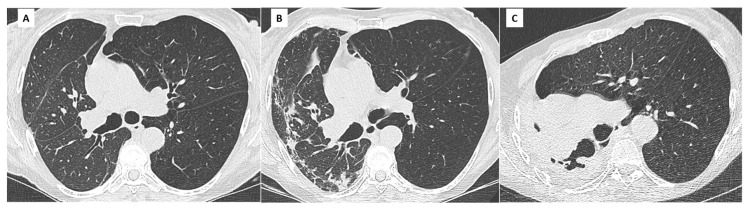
Unilateral RAS in a 61-year-old woman, who underwent right LT for α1-antitrypsin deficiency. (A): Baseline CT scan. Note the diffuse hypo attenuation and hypoperfusion of the left native lung, compared to the right allograft. An adapted normal FEV1 must be defined in this patient, taking into account the severe obstruction from the left lung; (B) Diagnosis of CLAD, with concomitant apparition of subpleural interstitial opacities in the right lung; (C) 3 years later, the allograft has been replaced by dense fibrosis and is totally collapsed. There is marked-over expansion of the left lung.
